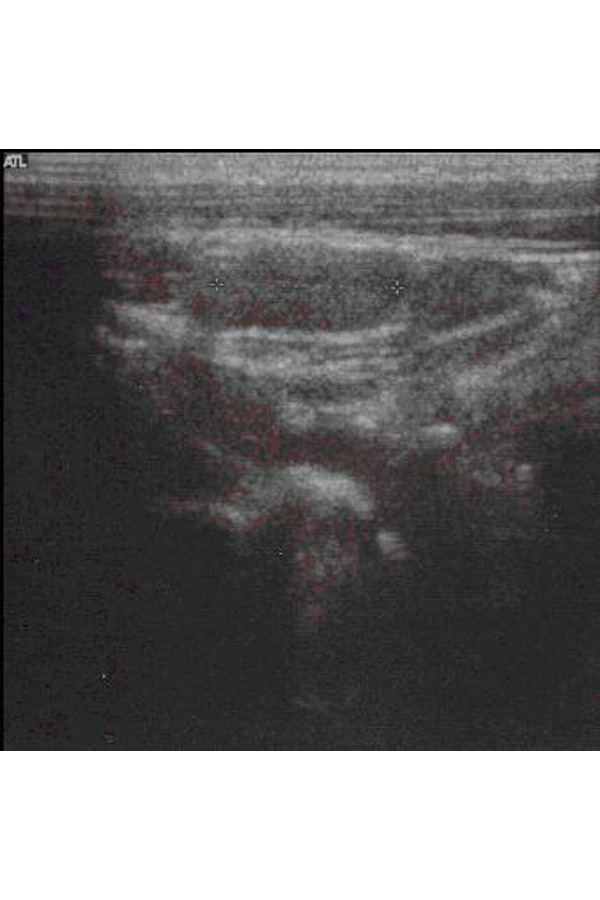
Clinical case 3. Ultrasound image of a nodule at the level of the right thyroid lobe of 21x13 mm, with internal calcifications.
